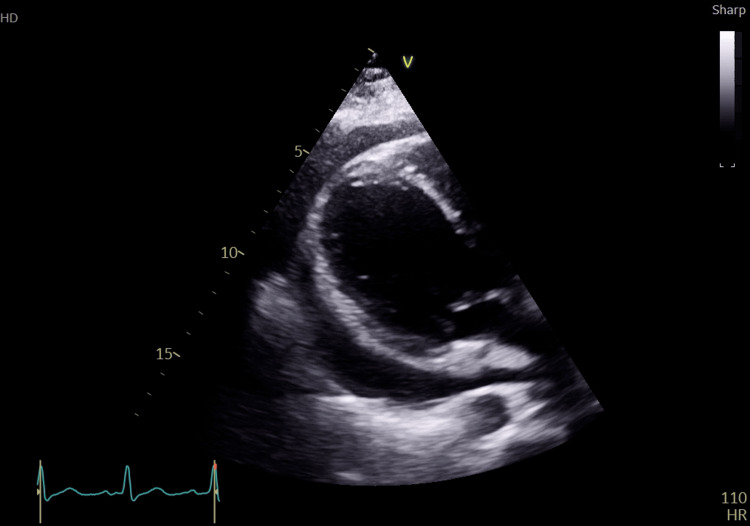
Echocardiography showing pericardial effusion
